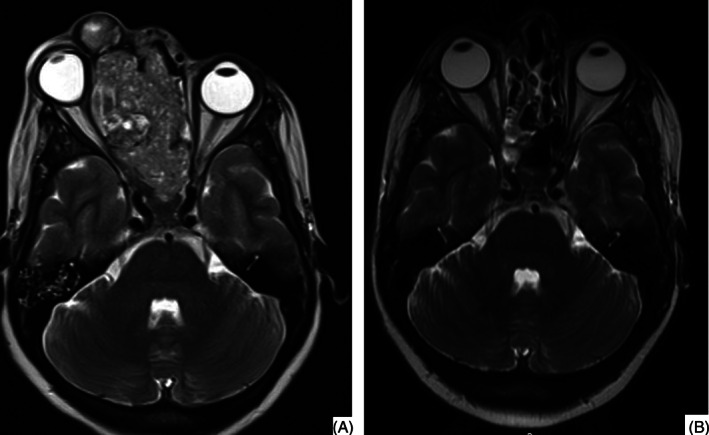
T2‐weighted images of the MRI. Panel A showing the tumor mass involving para‐nasal sinuses and causing proptosis; Panel B showing the reduction in the tumor size and resolution of proptosis after neoadjuvant chemotherapy
